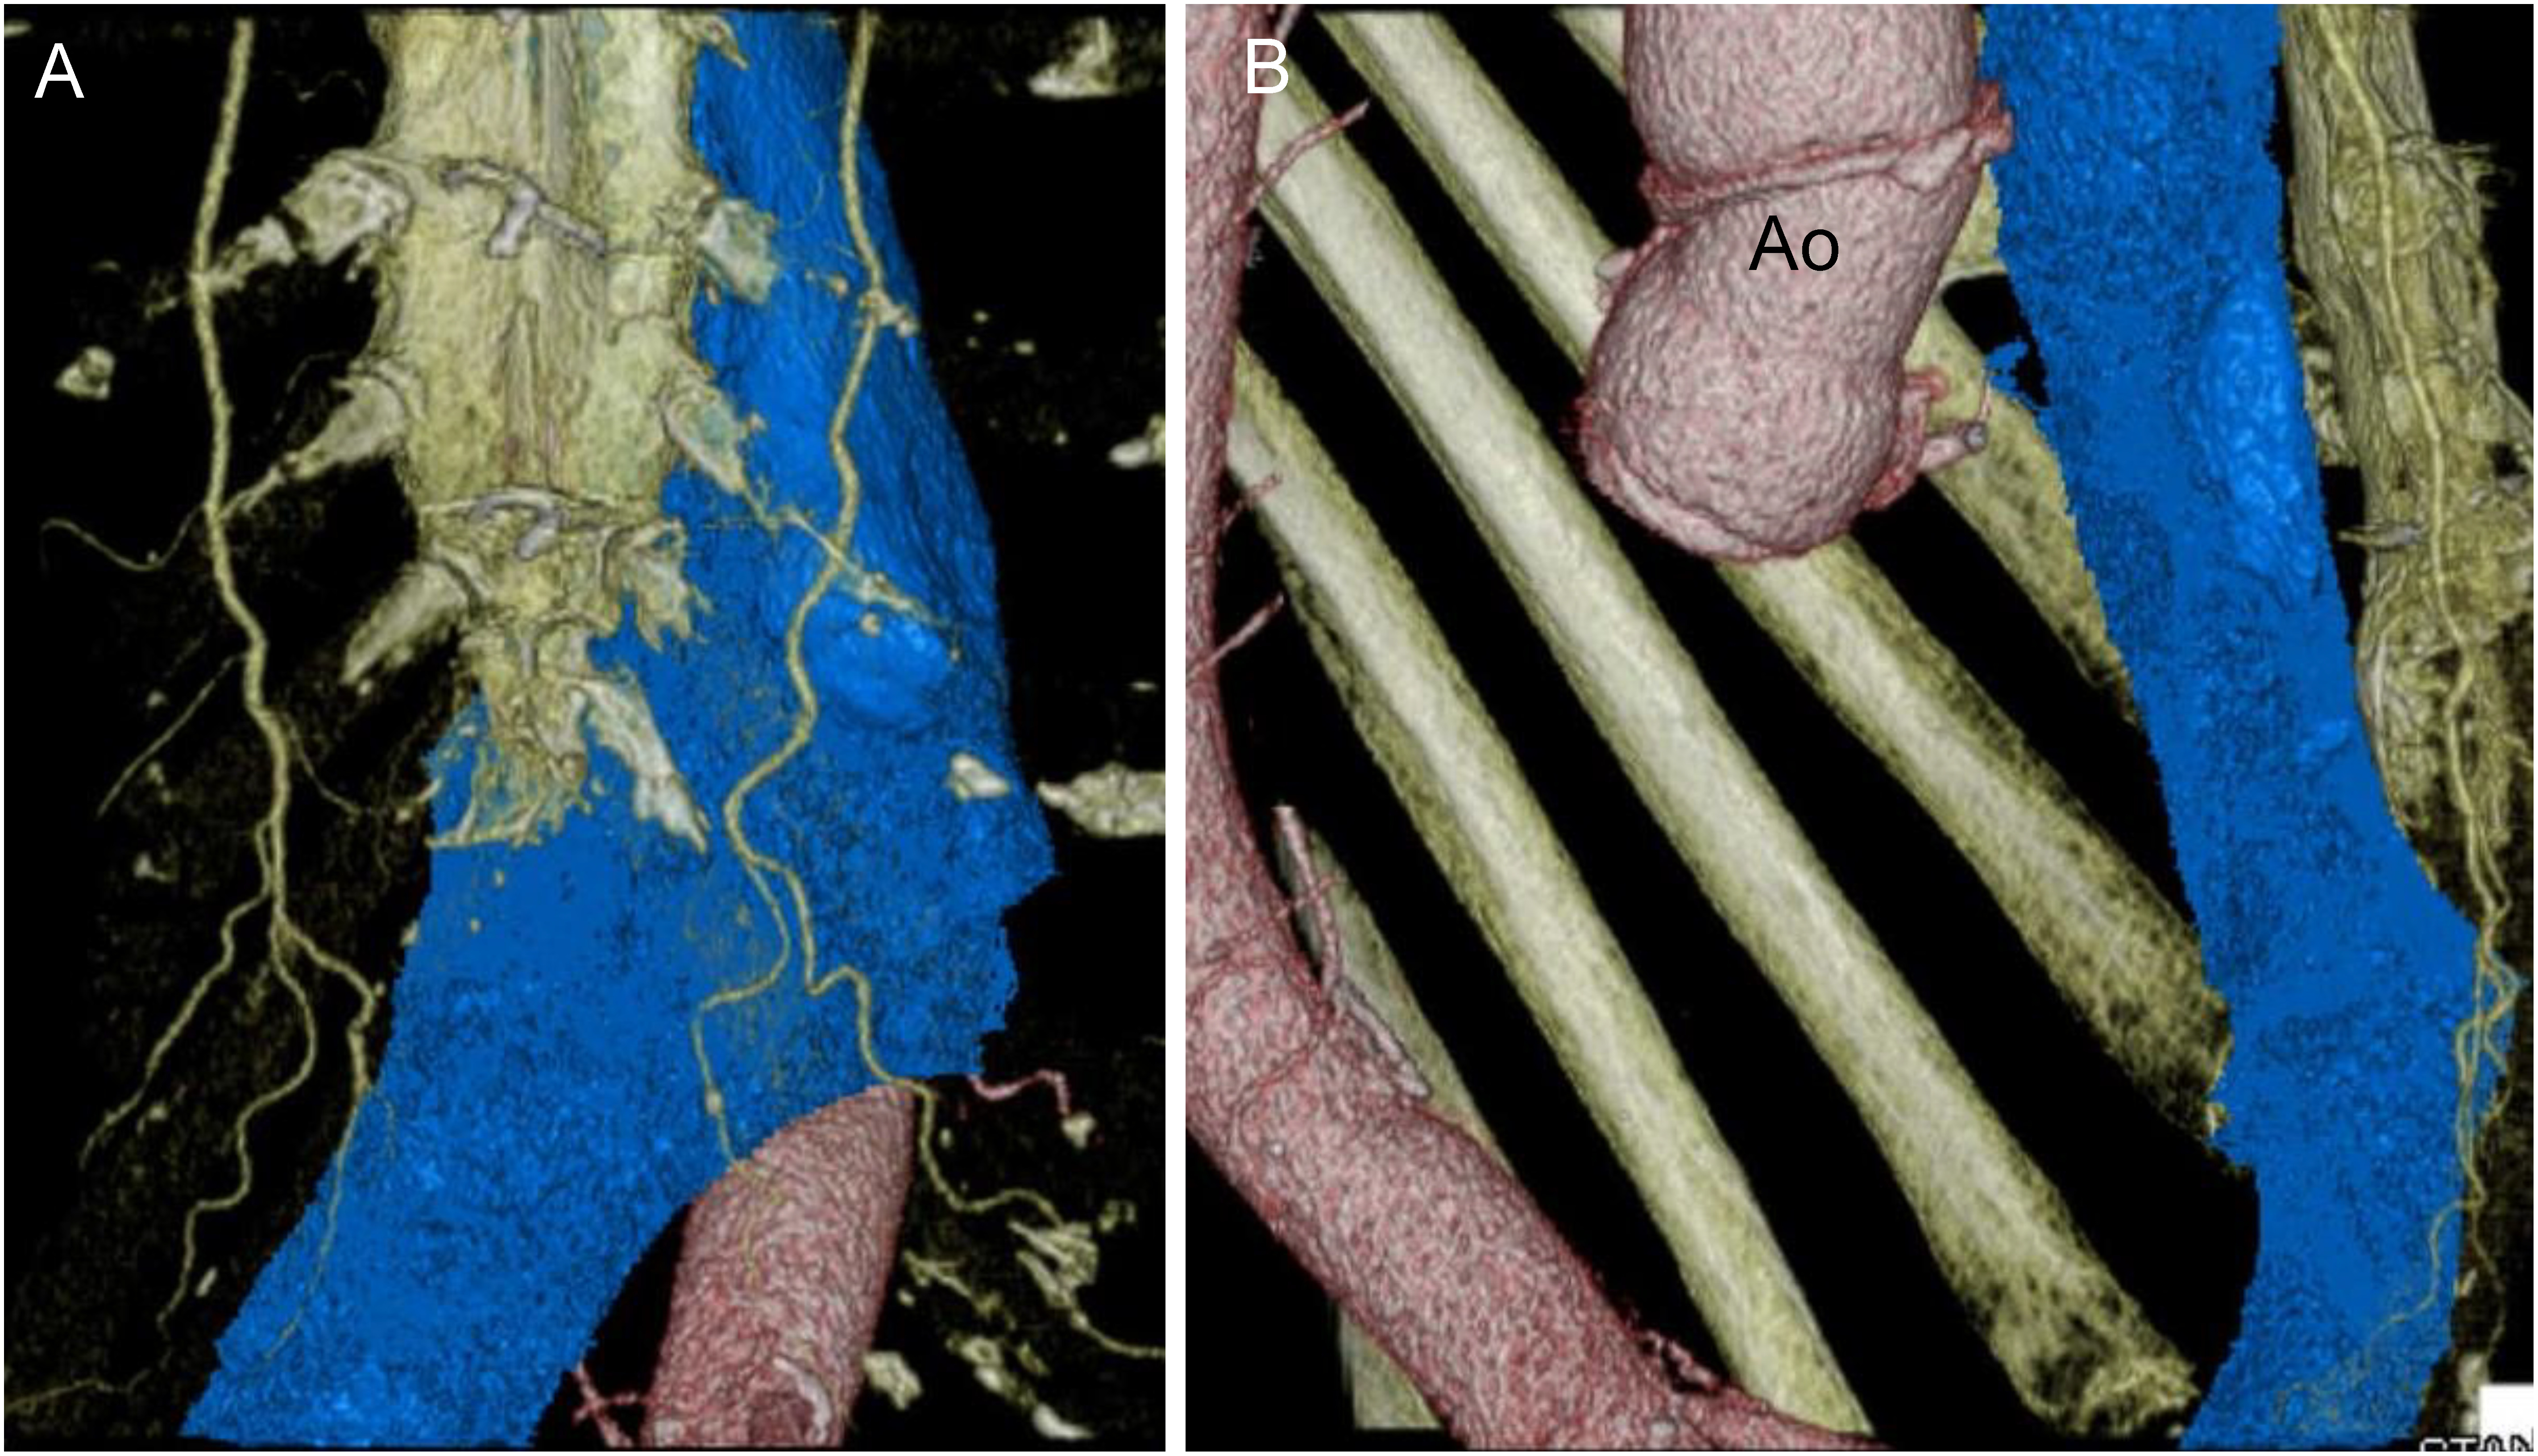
Fig. 3 Postoperative three-dimensional computed tomography. The aortic root was replaced without damage of the gastric tube.
